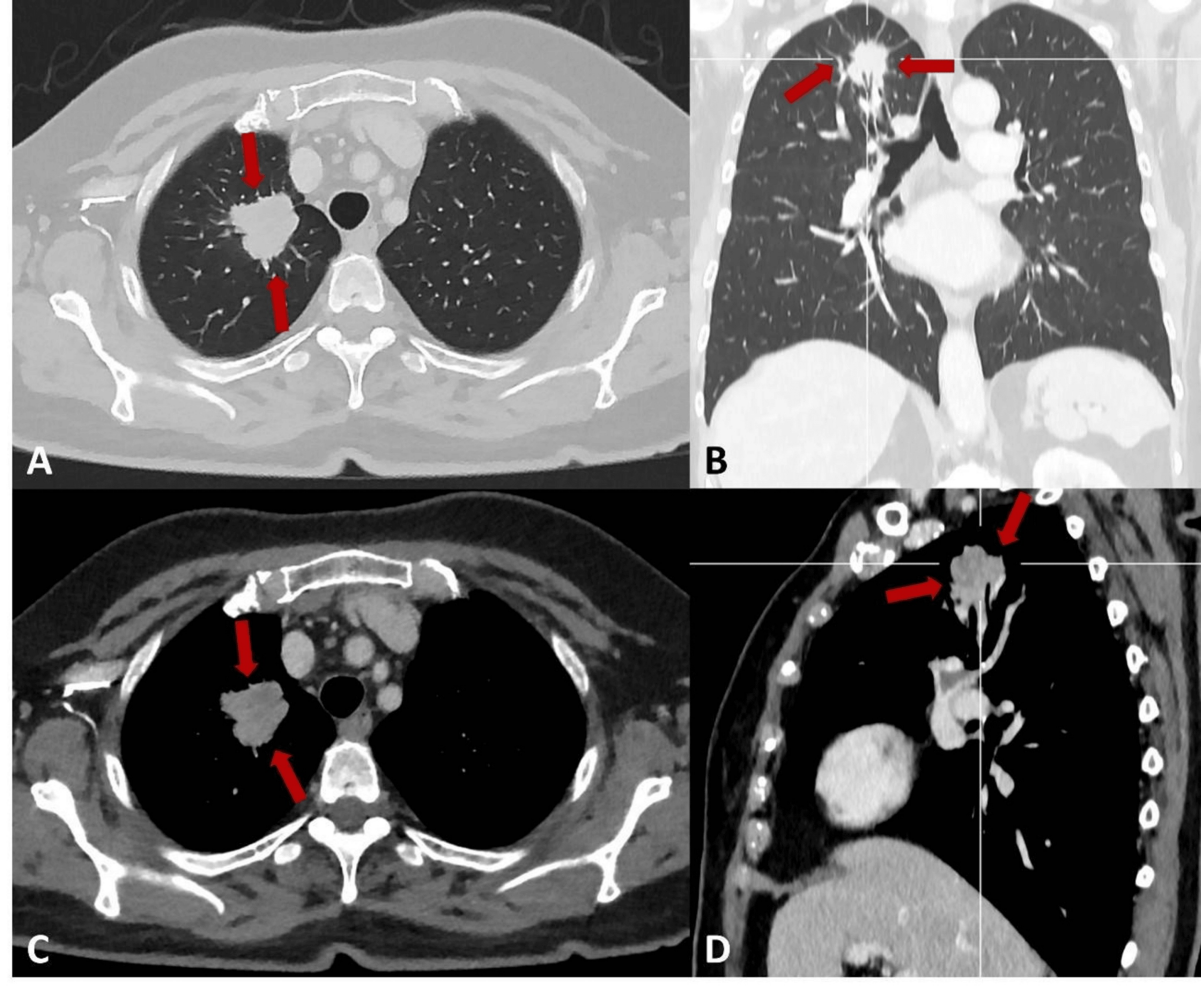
Chest CT showing a solid nodule with spiculated contours (red arrow) in the apical segment of the right upper lobe. (A) Lung window axial plane. (B) Lung window coronal plane. (C) Soft tissue window with contrast axial plane. (D) Soft tissue window with contrast sagittal plane. CT: computed tomography
